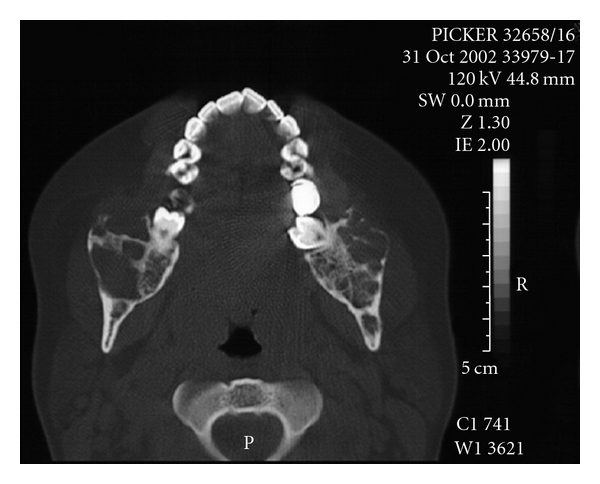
Axial CT scan revealing large hypoattenuated heterogenous image with aspect of expansive and invasive, osteolytic, multilocular lesion with perforation of the cortical bone at the angle and ramus of the mandible (Gomes et al. [6]).
